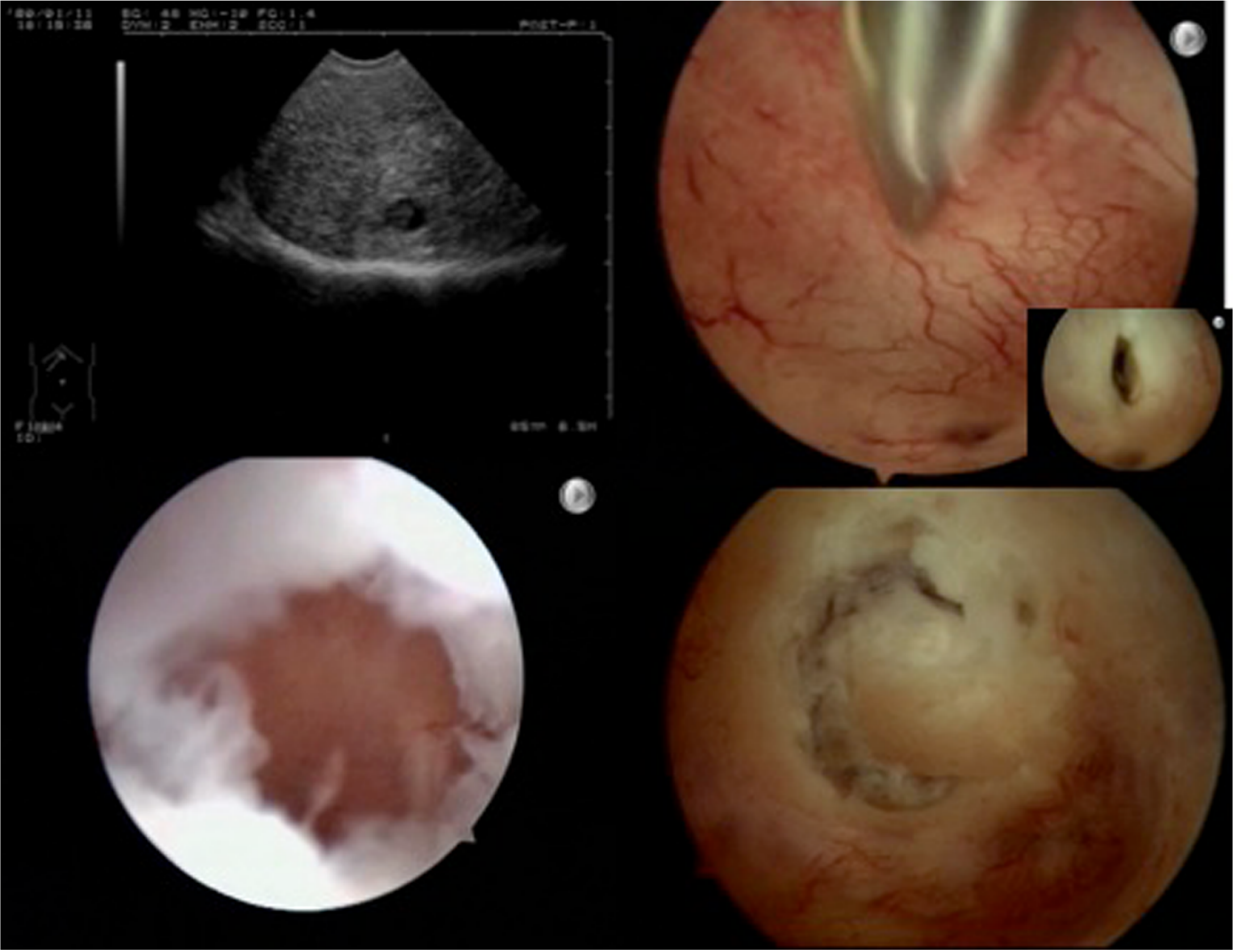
Case no. 1. a Cystic adenomyotic lesion at transvaginal ultrasound. b Abnormal vascularization and detail of the opened cyst after outflow of the brownish fluid. c Inside view of the cystic structure. d Dissection of cyst using 5-Fr scissors
